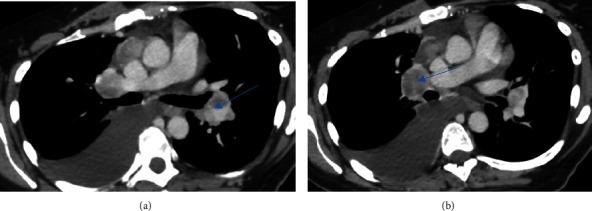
CT chest. (a) Confluent prevascular nodal mass measuring 4.1 × 2.4 cm. (b) Right hilar lymph node measuring 2.5 × 3.1 cm.
